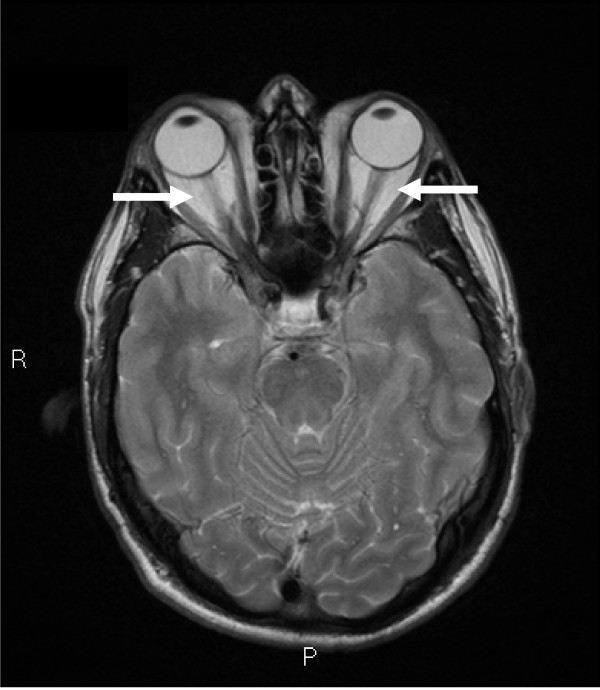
Magnetic Resonance Imaging of the orbits, showing congestion of the retro-orbital space and enlargement of the extraocular muscles *(arrows)*, consistent with the diagnosis of Graves' Ophthalmopathy.
